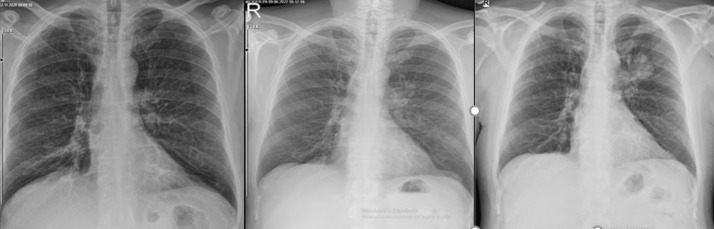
Sequential chest X-rays of a patient taken 3 years prior (left), 15 months prior (middle), and at the time of diagnosis (right).
